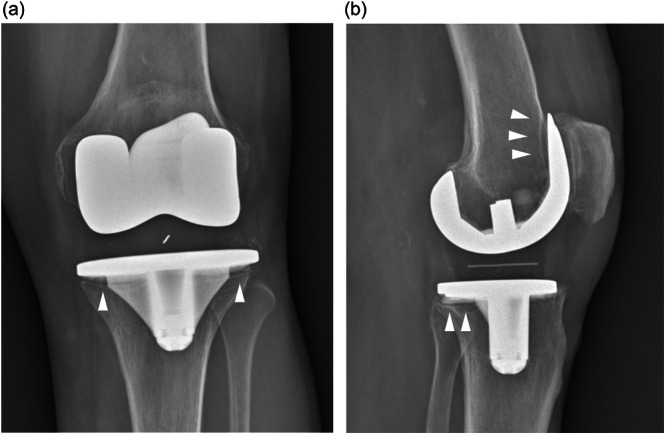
Anterior‐posterior (a) and lateral (b) radiographs of the knee taken at 10‐year follow‐up showing periprosthetic radiolucent lines (white arrowheads) around knee implant with VEPE tibial insert. VEPE, vitamin E‐stabilized polyethylene.
